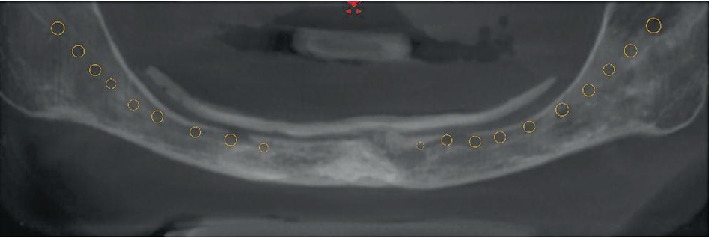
Panoramic view of the CBCT scan. The mandibular canal is traced. The opening of a neurovascular bundle under soft tissue is noted.
